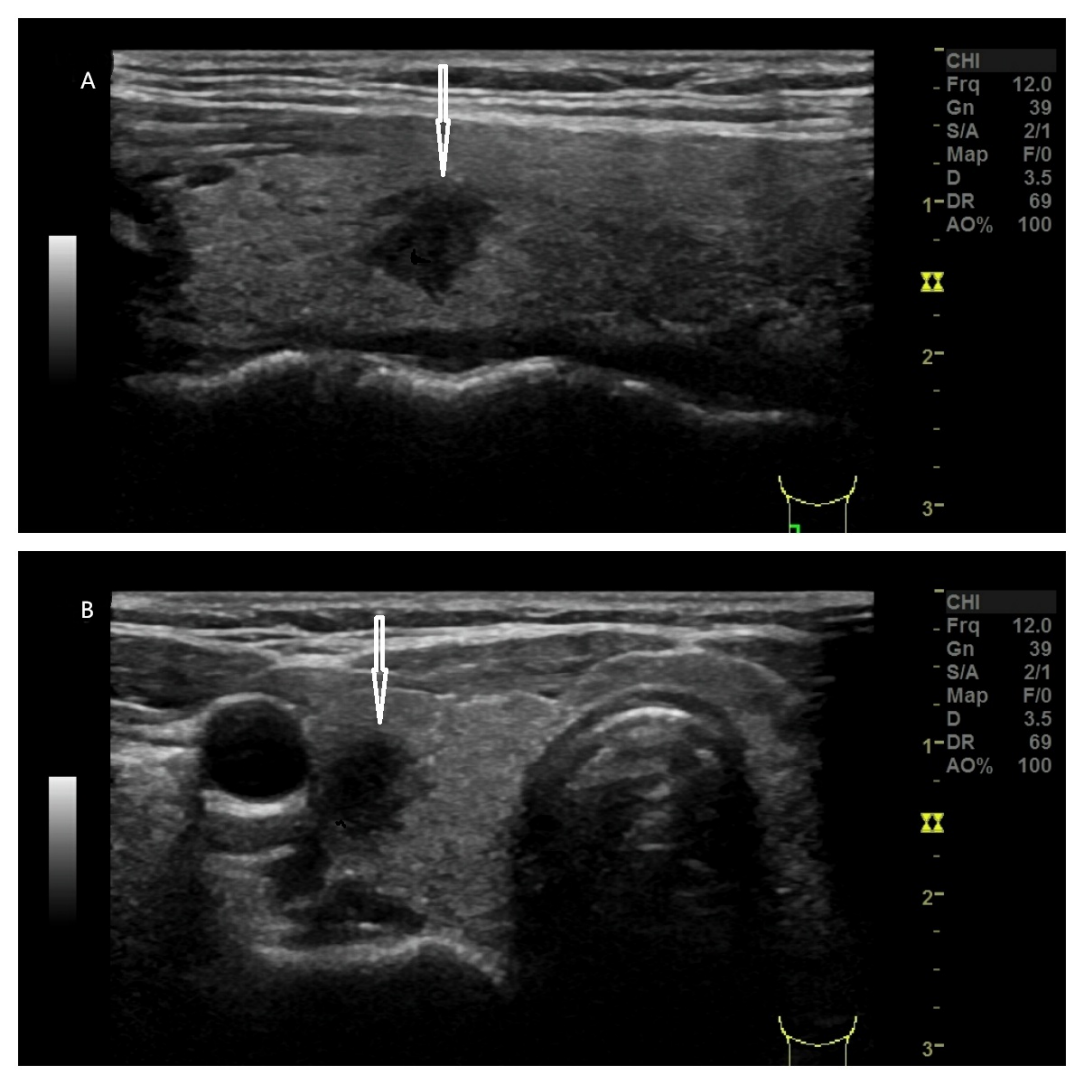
(A–B) A 37-year-old female with a pathologically confirmed thyroid nodule (papillary carcinoma). Ultrasound demonstrates a markedly hypoechoic nodule (arrows indicate) in the right thyroid lobe with the following characteristics: irregular shape (with taller-than-wide), entirely solid composition, two fine punctate hyperechoic foci, partially discernible margins, and dimensions of 8.1 × 6.2 × 7.4 mm. No posterior acoustic features observed. Classification: classical C-TIRADS 5, modified C-TIRADS 5, K-TIRADS 5.
